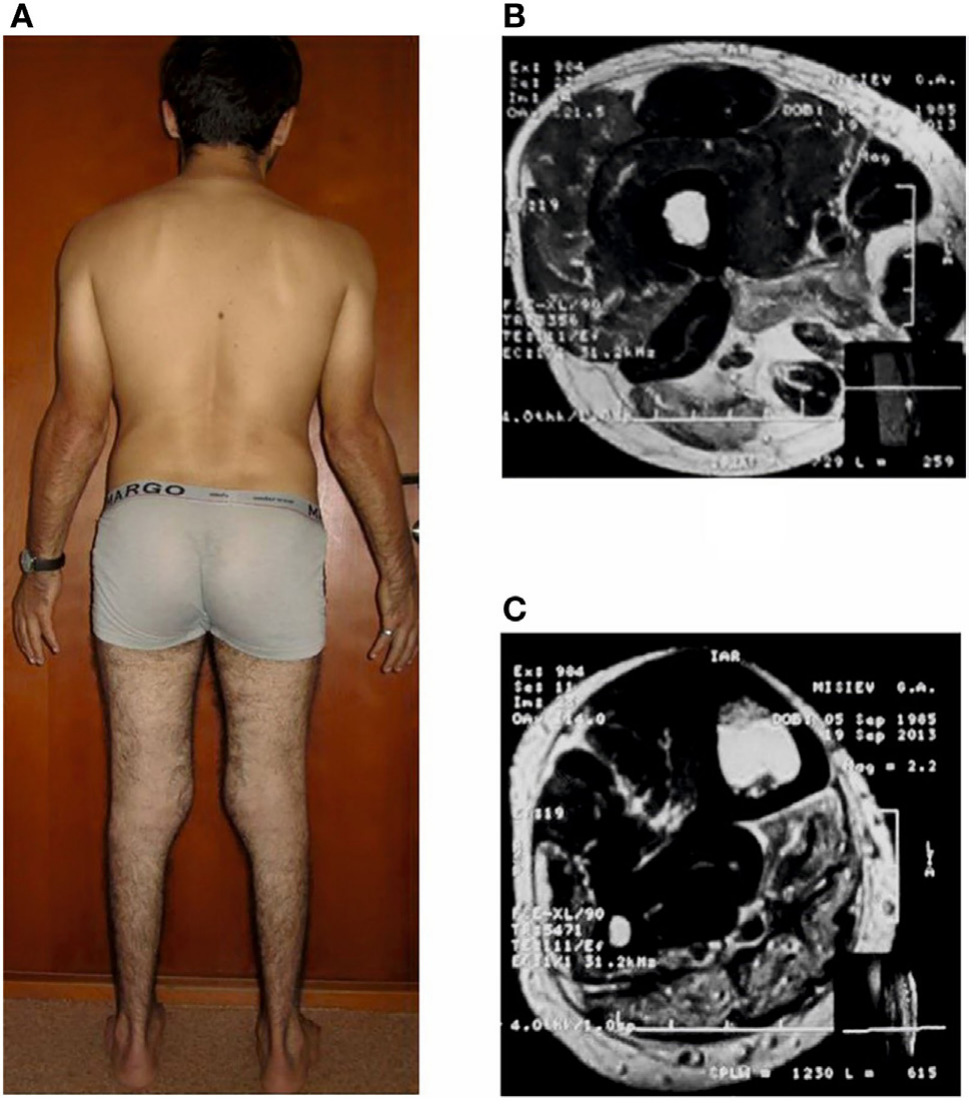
Patient C-1*, age 28 with distal Miyoshi myopathy (A) and magnetic resonance images (T2-WI) at the level of the middle third of the thigh (B) and lower leg (C).
